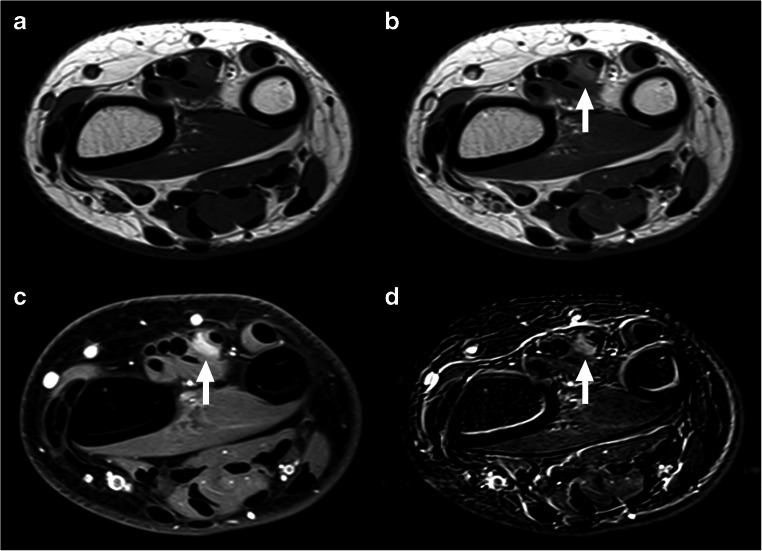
Example of true-positive MRI scan in a 35-year-old woman with synovial sarcoma who had undergone a Whoops procedure at the dorsal side of the right wrist. Axial T1-weighted (a), gadolinium chelate-enhanced T1-weighted (b), fat-suppressed gadolinium chelate-enhanced T1-weighted (c), and subtraction gadolinium chelate-enhanced (d) images at the level of the right wrist. There is a contrast-enhancing nodular lesion adjacent to the extensor digiti minimi tendon (arrows), which was interpreted as deep-seated residual tumor, and confirmed by pathological examination after second surgery
